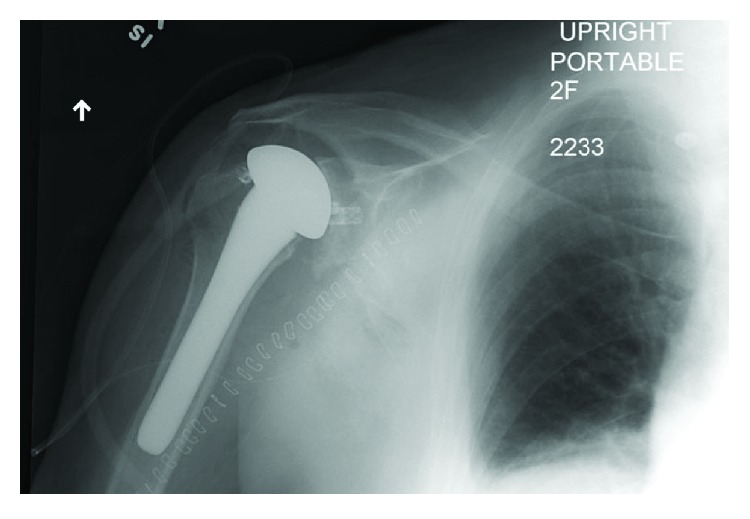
Postoperative X-ray, day of primary TSA.
